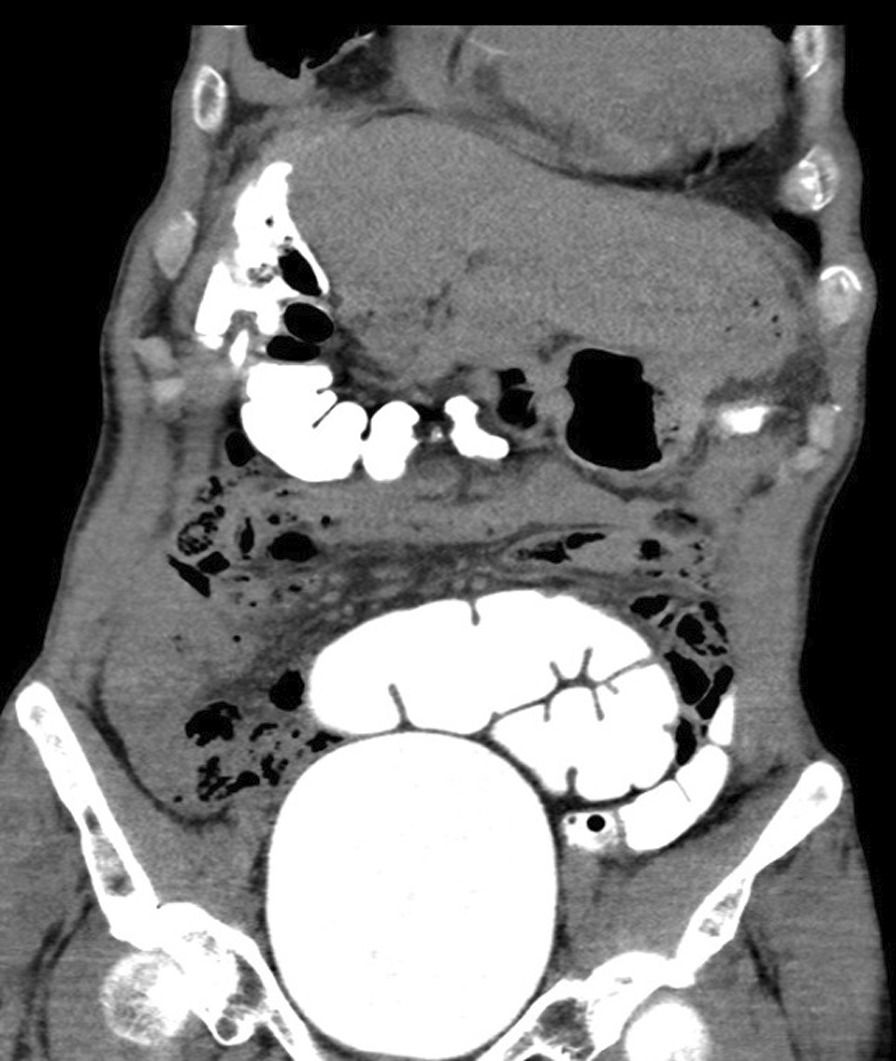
Computed tomography (CT) image confirming a colopleural fistula with contrast outlining a fistula tract between the colon and right lower lobe
